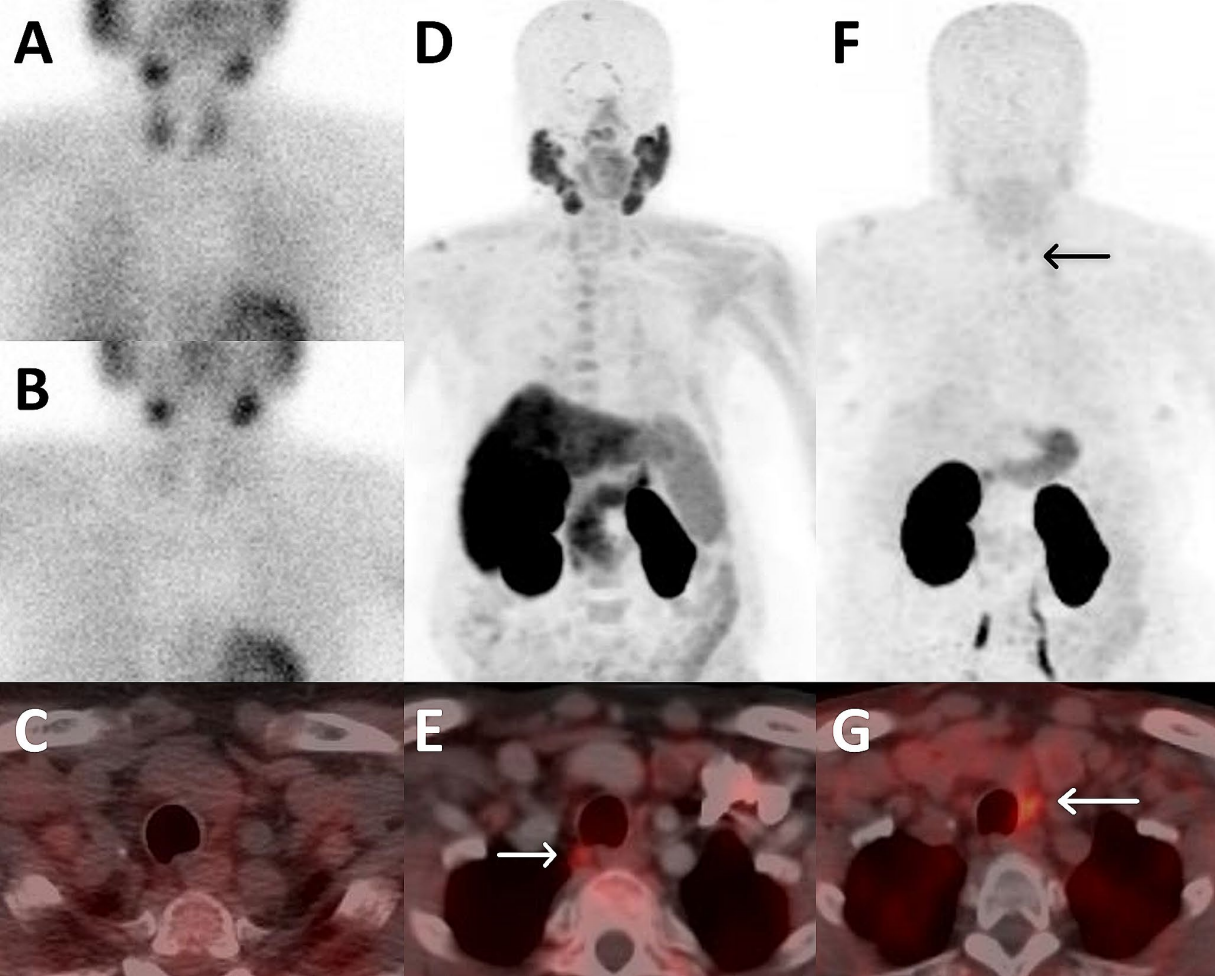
A 65-year-old woman with incidental PHPT (serum PTH: 108 ng/l Calcium: 11.5 mg/dL) underwent initial MIBI scan. However, both early and delayed planar (A, B) and SPECT/CT (C) images did not exhibit tracer uptake. Subsequent assessment with [^18^F]fluorocholine PET/CT (D) revealed uptake in an upper right paratracheal lesion (E). Additionally, ^68^Ga-Trivehexin PET/CT demonstrated uptake (F) in a lesion posterior to the left lower thyroid lobe on axial images (G). Further investigation through PTH-WO confirmed the false-positive uptake observed on [^18^F]fluorocholine PET/CT , while accurately identifying the uptake observed on ^68^Ga-Trivehexin PET/CT as indicative of a parathyroid lesion
